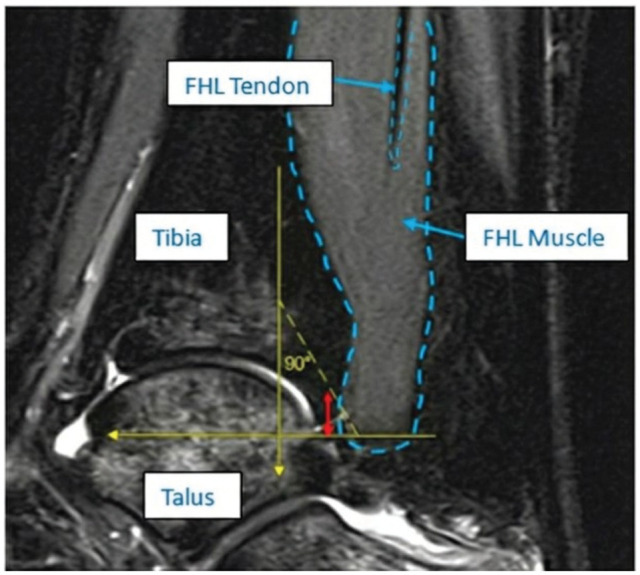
Mid-sagittal T1-weighted MRI shows a 2-mm absolute MTJ distance (red arrow) on the right ankle of a 15-year-old female with os trigonum syndrome. Note that the label "FHL Tendon" points to the intramuscular portion of the tendon extending proximally within the muscle belly. The absolute MTJ distance is measured by the red arrow, representing the vertical distance starting from the level of the posteroinferior corner of the distal tibia (indicated by the horizontal yellow reference line) up to the distal-most point of the MTJ, parallel to the tibial shaft (vertical, yellow line). FHL, flexor hallucis longus; MRI, magnetic resonance imaging; MTJ, musculotendinous junction.
